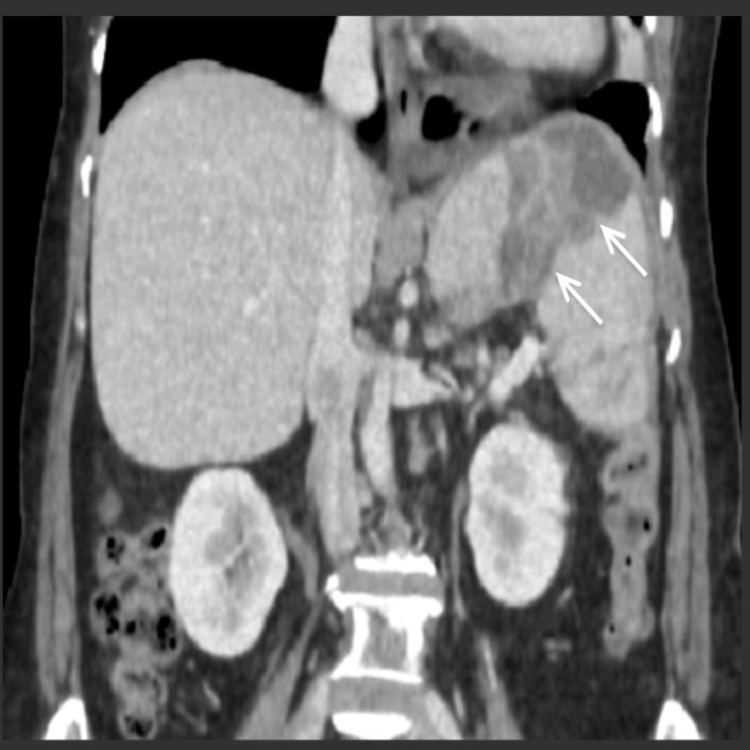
CECT abdomen showing extension of the splenic abscess into the sub-diaphragmatic region Capsular thinning and perisplenic inflammatory changes observed, which raised concern for impending rupture; Contrast-Enhanced Computed Tomography
